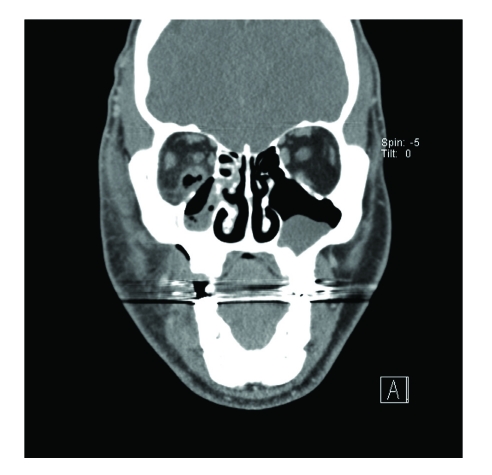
Coronal CT scan (admission) shows orbital emphysema continuous with the right inferior rectus and thickening of the adjacent maxillary sinus
